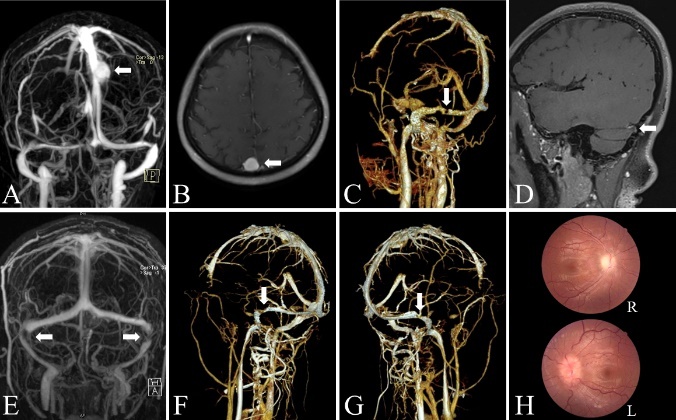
Imaging findings in patients with CVS stenosis. (A-B) Large meningioma-induced superior sagittal sinus stenosis seen with CE-MRV and with MRI enhancement. In CE-MRV, a well-defined meningioma caused confined stenosis of the superior sagittal sinus (A). The meningioma demonstrated hyperintense signal intensity with MRI enhancement, and caused significant compression of the superior sagittal sinus (B). (C-D) Large arachnoid granulation-induced transverse sinus stenosis seen with CTV and MRBTI. Severe stenosis of the left transverse sinus was observed with CTV (C). MRBTI showed a large arachnoid granulation in the left transverse sinus (D). (E-H) Severe bilateral transverse sinus stenosis seen with MRV and CTV. Severe stenosis of the bilateral transverse sinuses was observed using MRV (E) and CTV (F-G). Fundus examination showed bilateral papilledema (Frisen grade Ⅳ in the left eye and Frisen grade II in the right eye) (H).
